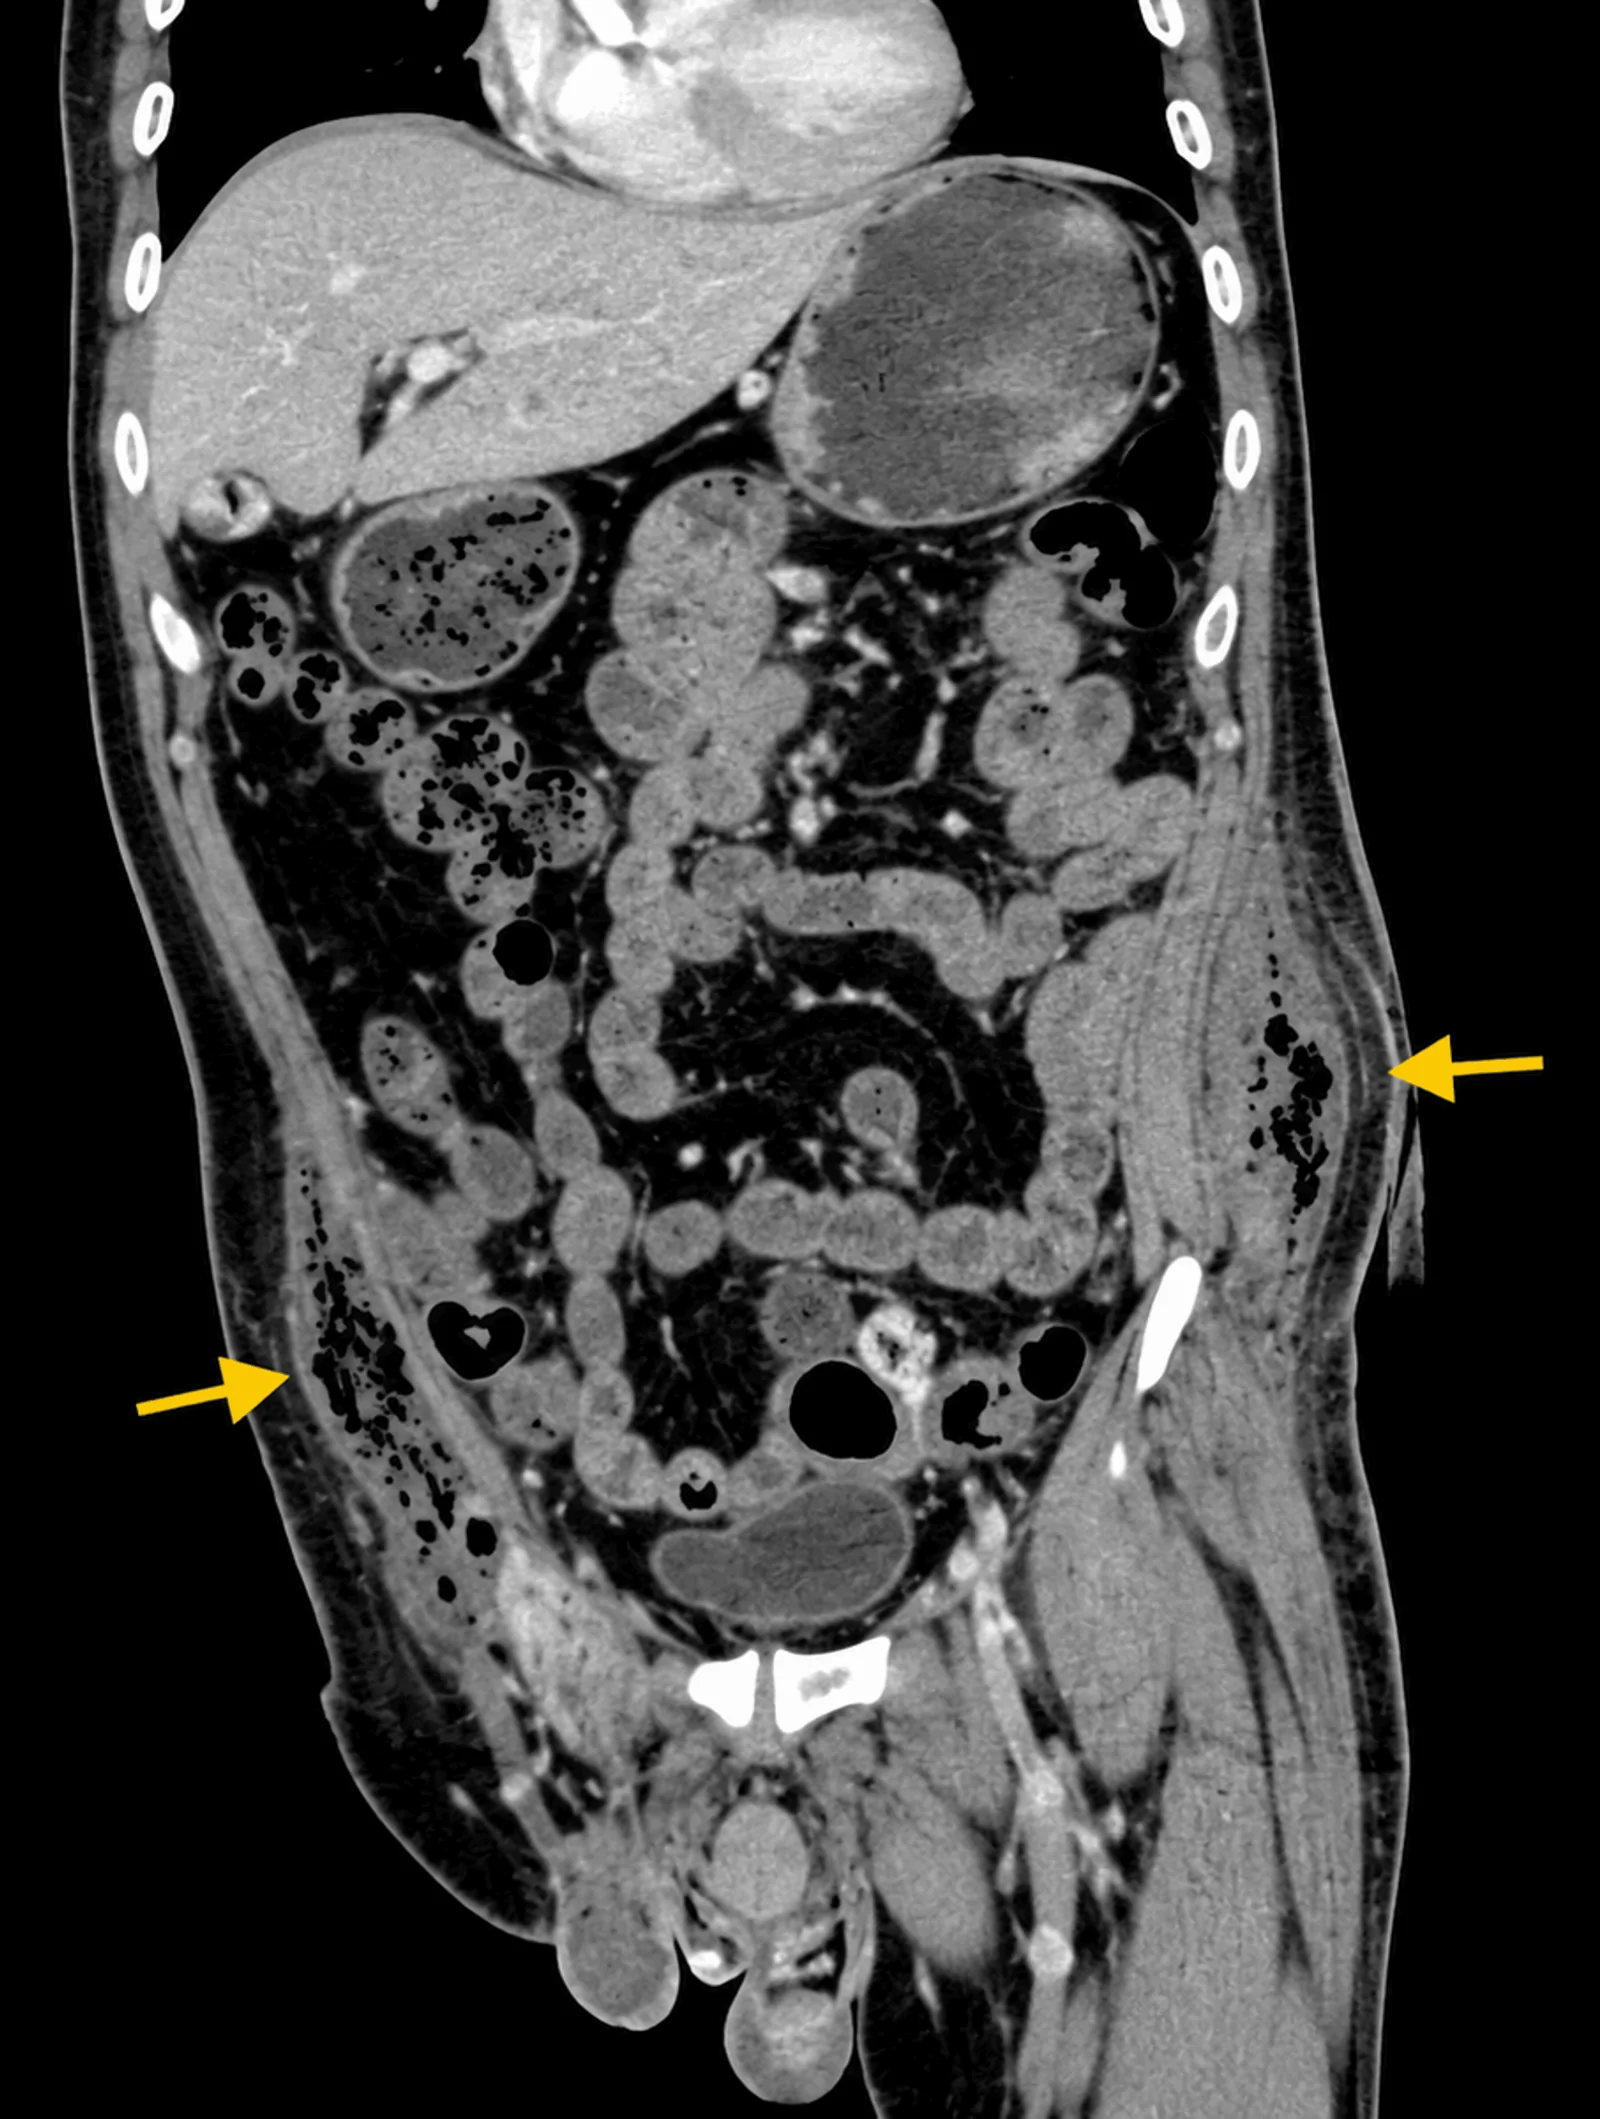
Repeat CT of the abdomen and pelvis Contrast-enhanced coronal CT image demonstrating bilateral lower abdominal wall soft-tissue fluid and gas collections (arrows), concerning for abscesses. Image-guided drainage was subsequently performed. CT: computed tomography
